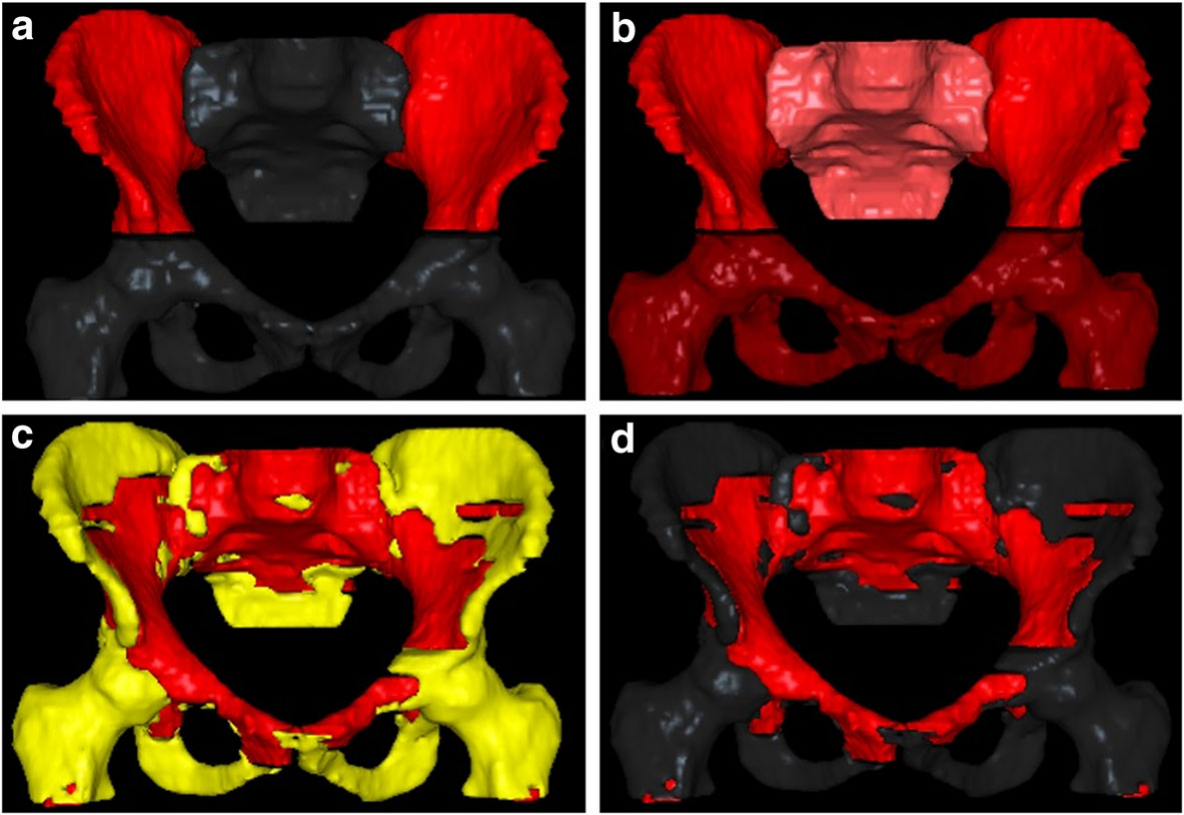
Visual representation of the 4 planning approaches. Bone marrow is represented in red. Optimization was addressed to iliac crest in Plan A (a), the whole pelvic bones defined as external osseous contour in Plan B (b), active (red) and inactive (yellow) bone marrow as defined with ^18^FDG-PET (c) with a higher priority for active and a lower for inactive, active bone marrow only as defined with ^18^FDG-PET (d)
